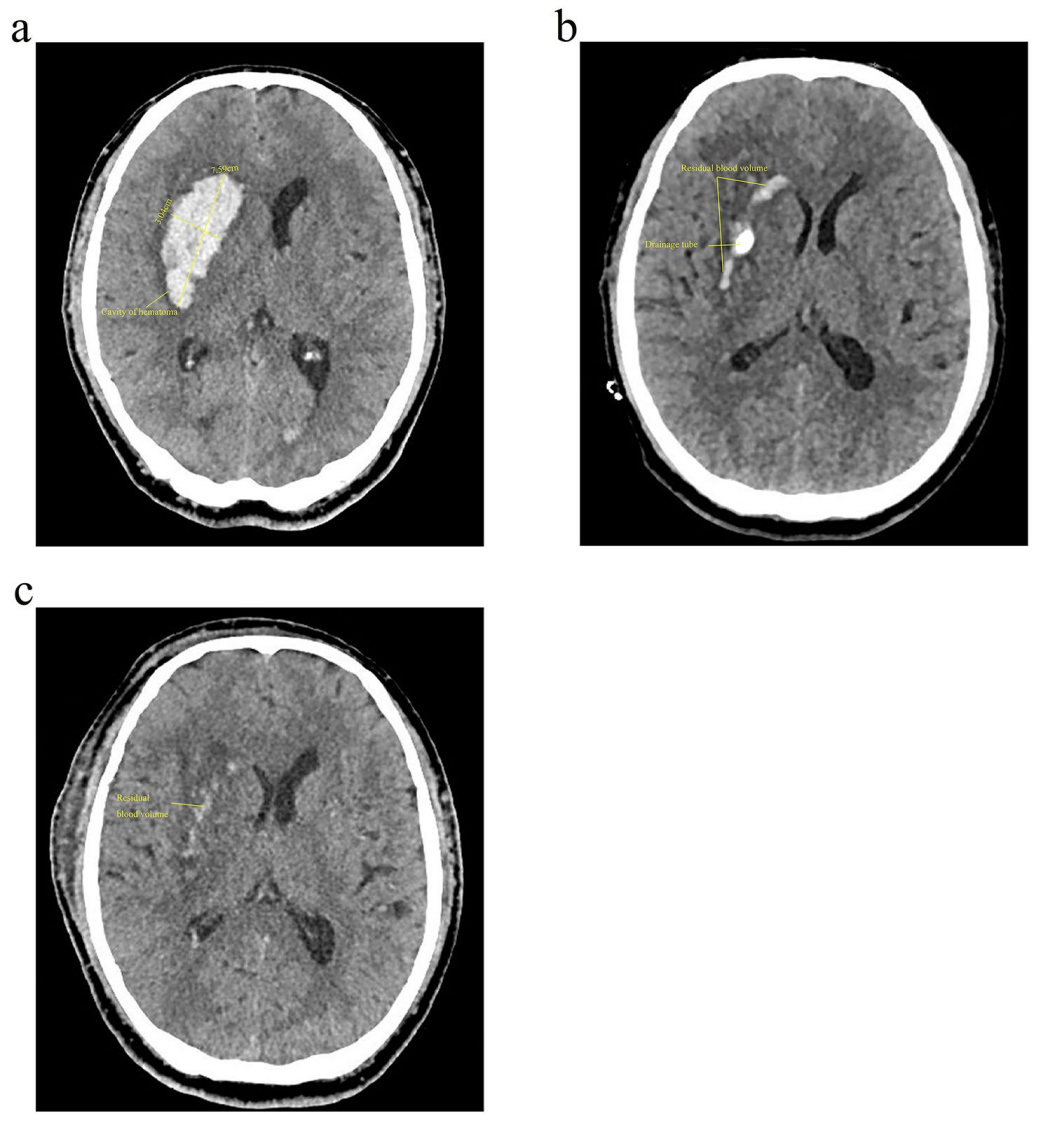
A 64-year-old man experienced headache for 3 h and underwent neuronavigation-assisted intracerebral hematoma puncture and drainage of cerebral hematoma. (a) Preoperative CT, hematoma volume 33.701 ml; (b) CT before extubation, remaining hematoma volume 5.762 ml; (c) CT after extubation, remaining hematoma volume 1.935 ml
